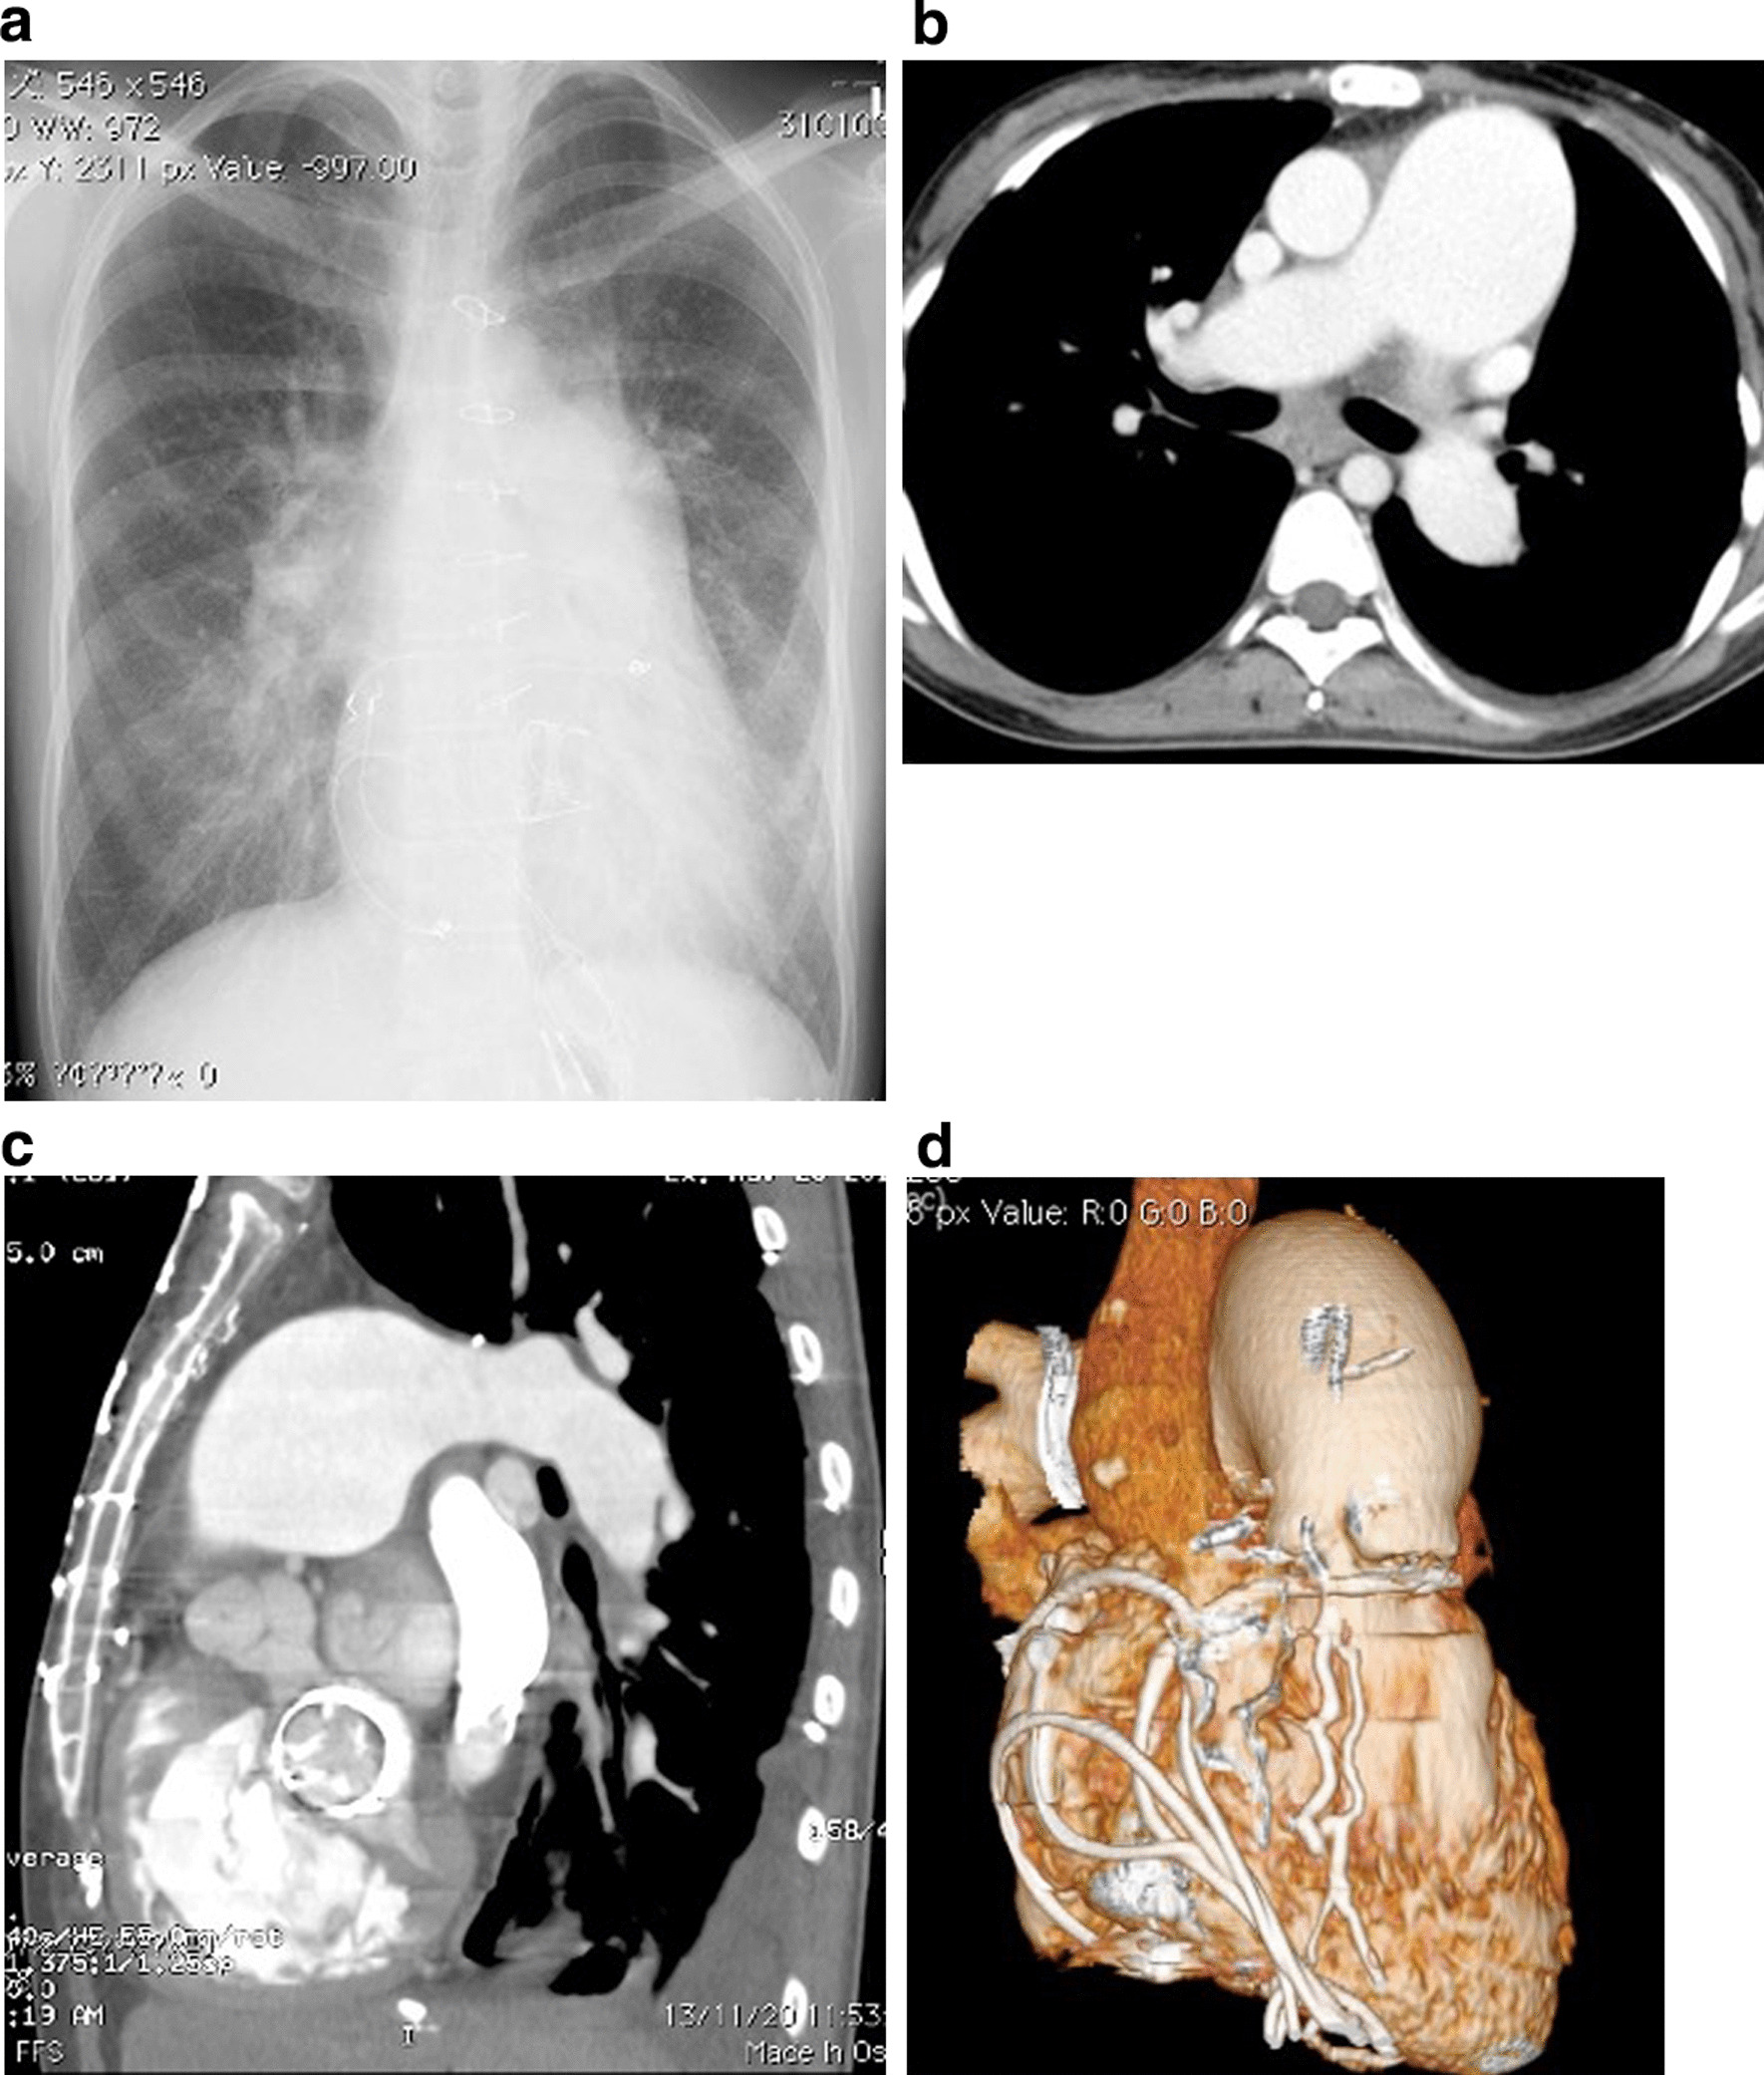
a. Preoperative chest radiograph shows cardiomegaly involving the right cavities and notable enlargement of the pulmonary artery. b Preoperative computed tomography of the lung shows notable enlargement of the pulmonary artery. c Preoperative computed tomography of the lung shows notable enlargement of the pulmonary artery and severe calcification of the bioprosthesis. d Preoperative computed tomography of the lung shows notable enlargement of the pulmonary artery and severe adhesion of the previous pacing wires in the right atrium
